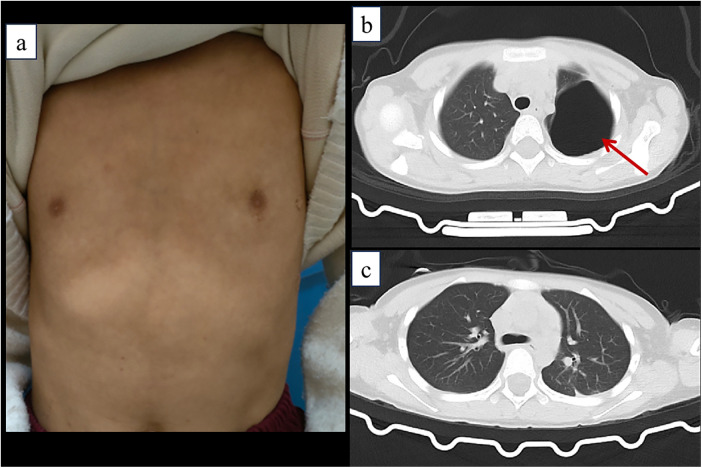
Chest examination of the patient. (a) Visual examination of the patient’ chest showed that the child's chest is flat with a depression in the lower part of the sternum and bilateral rib margins outward, consistent with the sign of funnel chest. (b) Enhanced CT scan showed a circular translucent lesion (red arrow) in the upper lobe of the left lung, with a thin wall and no lung markings inside. There were signs of adjacent lung tissue compression. (c) Follow-up chest CT after surgery showed a small cystic lucency with surrounding punctate calcifications at the left upper lobe surgical site and linear fibrotic changes in the left lower lobe.
